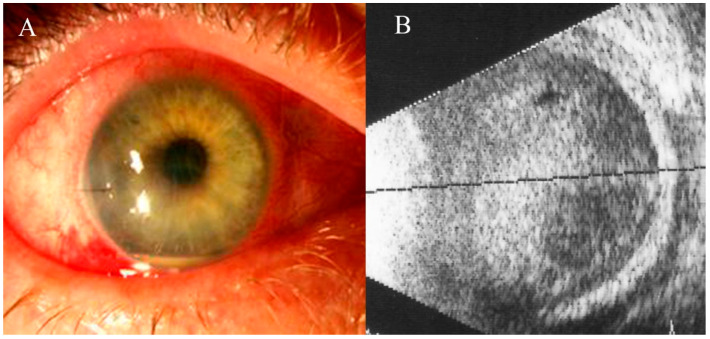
Prevalent signs of EE: (A) corneal decompensation and fibrin/hypopyon (B), ocular ultrasound scan showing infiltration of the vitreous cavity and retinochoroidal thickening.
